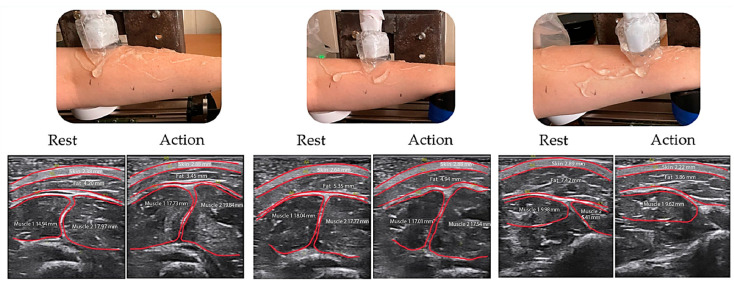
The location of the ultrasound sensor in the extreme left position, the maximum thickening of the myogaster and the extreme right position, and the corresponding ultrasound image of the anatomy of the forearm in some places and when performing an action.
